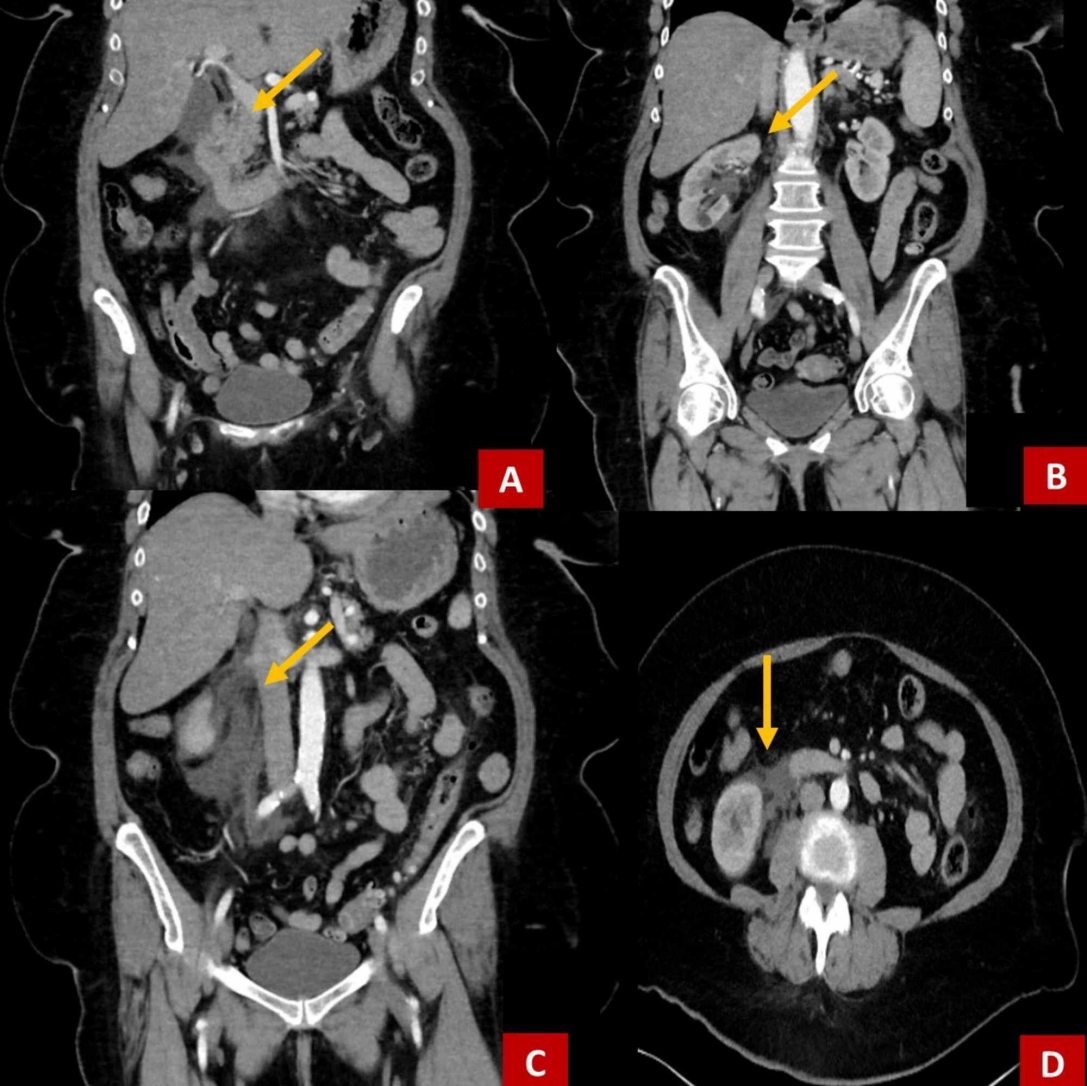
CT abdomen and pelvis coronal and transverse planes. Demonstrates fluid in the upper right quadrant near the kidney, stomach, and duodenum. Initial imaging was unclear of the etiology for this fluid. A,B,C: coronal plane, D: transverse plane.
